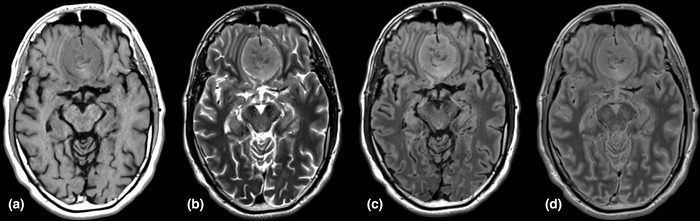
SyMRI acquired T1‐weighted (a), T2‐weighted (b), T2‐FLAIR‐weighted (c), and PD‐weighted (d) images on a parasagittal meningioma, reconstructed using the SyMRI software, Version 11.2 Ext. 2 (Synthetic MR AB, Linköping, Sweden)
